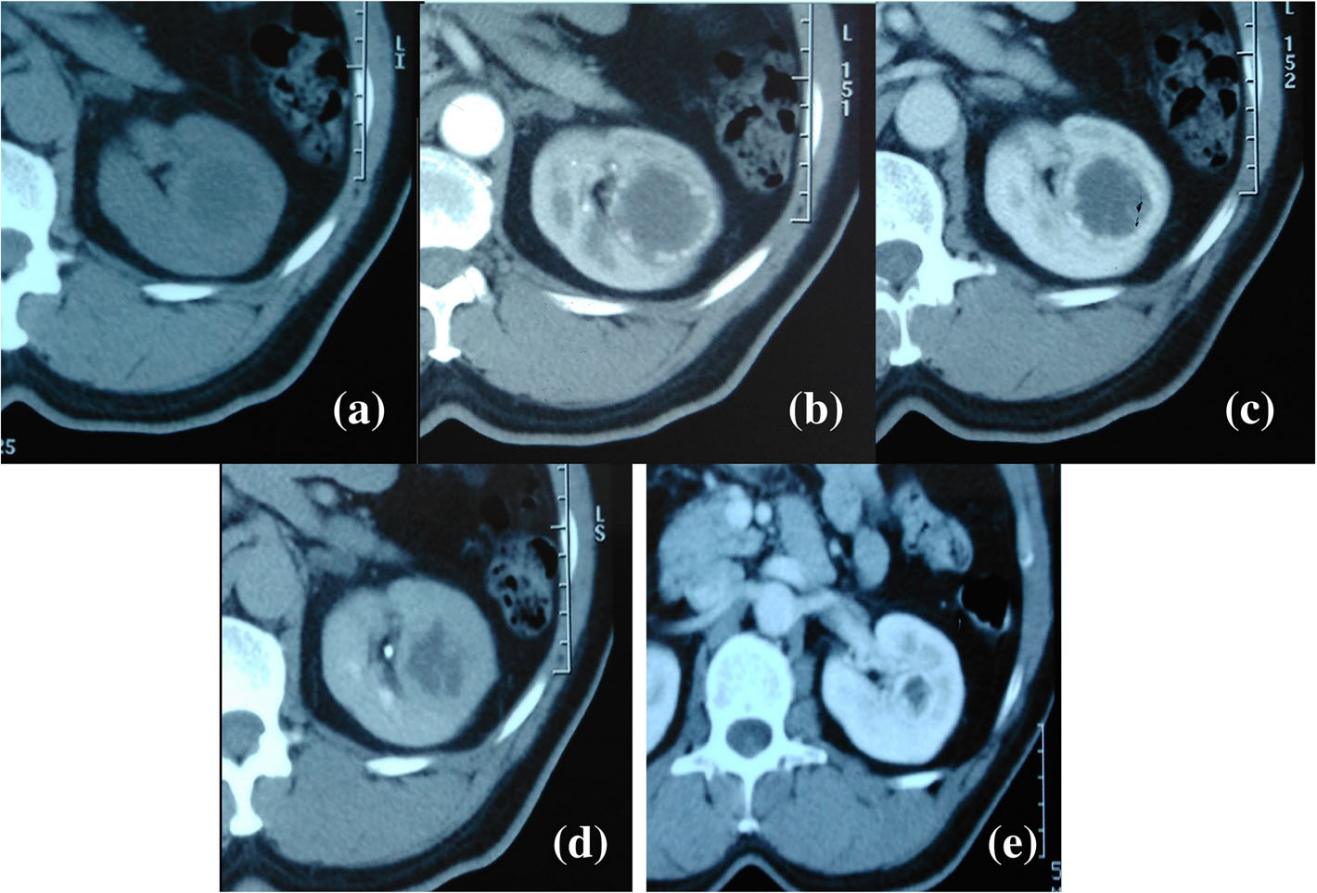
Computed tomography axial view of the tumor shows intense peripheral enhancement. a Non-enhanced computed tomography. b Arterial phase. c Portal phase. d Delayed phase. e The same lesion, 6 years ago
